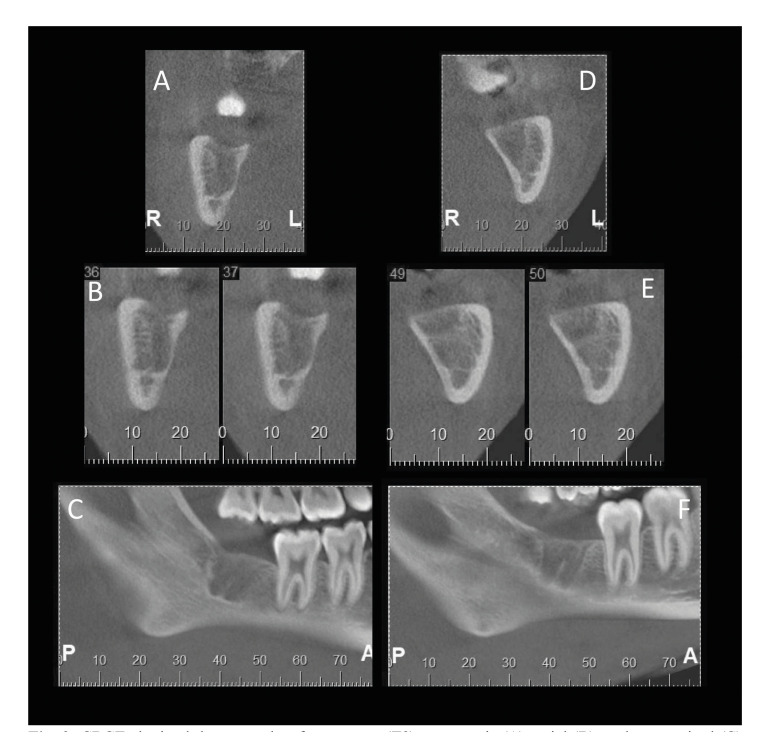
CBCT obtained three months after surgery (T3). panoramic (A), axial (B), and parasagittal (C) reconstructions showing the bone repair of a L-PRF socket and panoramic (D), axial (E), and parasagittal (F) reconstructions showing the bone repair of control a socket (same patient).
